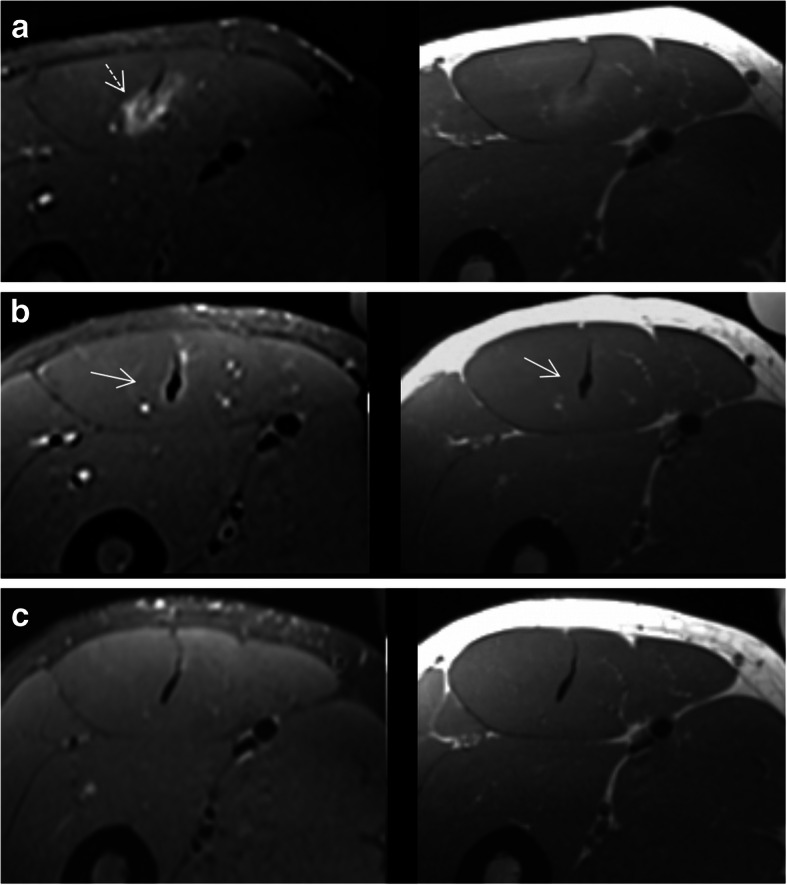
Evolution of healing by MRI over a course of 12 weeks: rectus femoris central myoconnective junction. Axial T1- and fluid-sensitive images at 1–2 weeks (a), 9 weeks (b), and 12 weeks (c) show progressive reduction of edema. a MR images show a soft fragmented callus (dashed arrow). b MR images show the callus organized and complete. It is hypertrophic, hypointense in fluid-sensitive image, and slightly heterogeneous in T1 (arrows). c MR images show a complete recovery of the tendon and peripheral musculature signal
